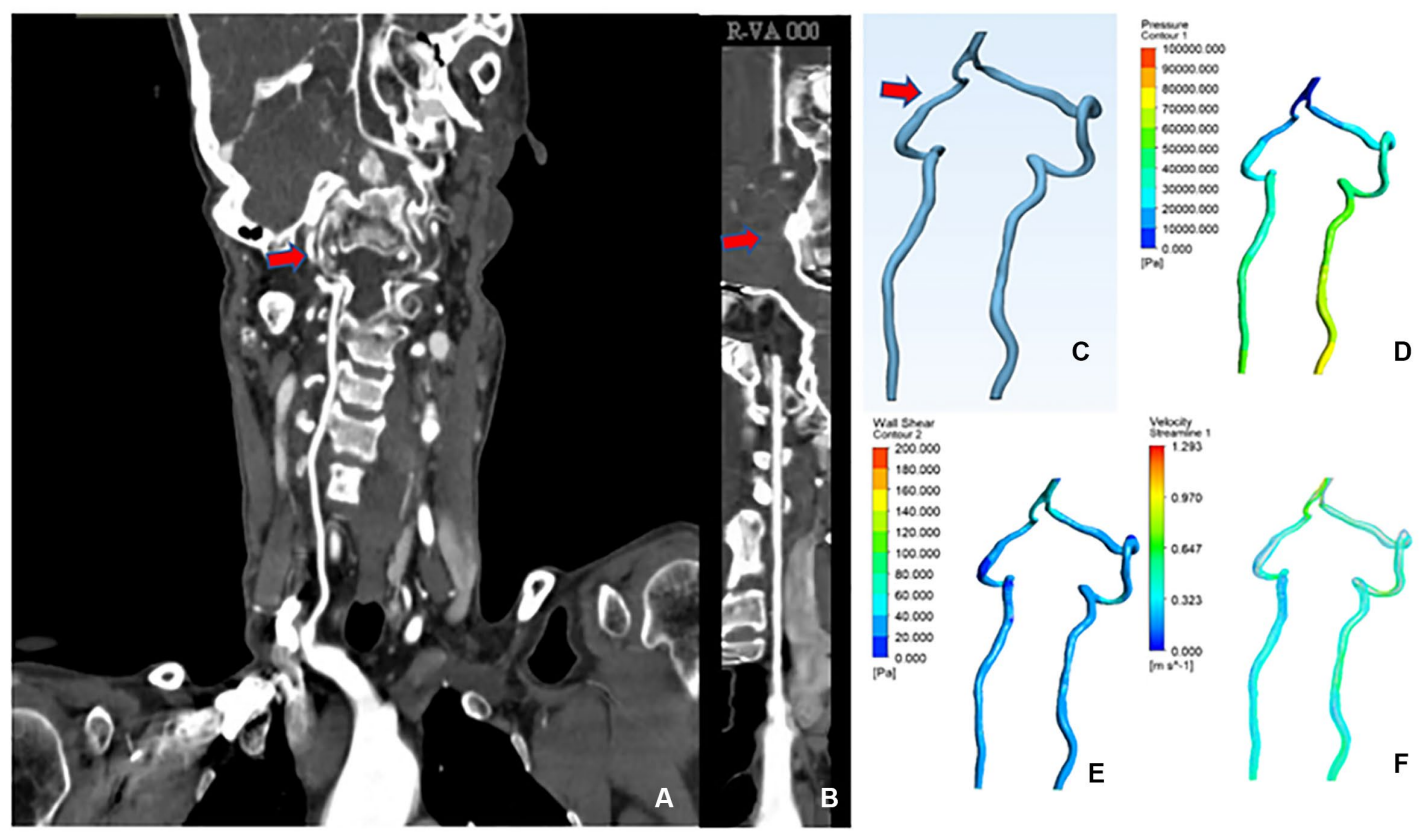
CTA and CFD images of vertebral artery in case 2. (A–F) The same as shown in Figure 4.
